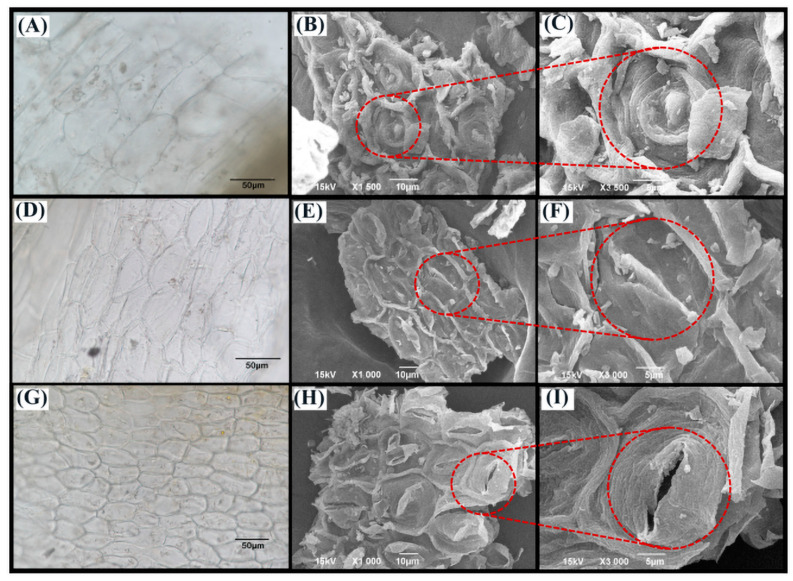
The OM and SEM images of AMF powder. (A–C) Maceration extraction, (D–F) reflux extraction, (G–I) ultrasonic extraction.
